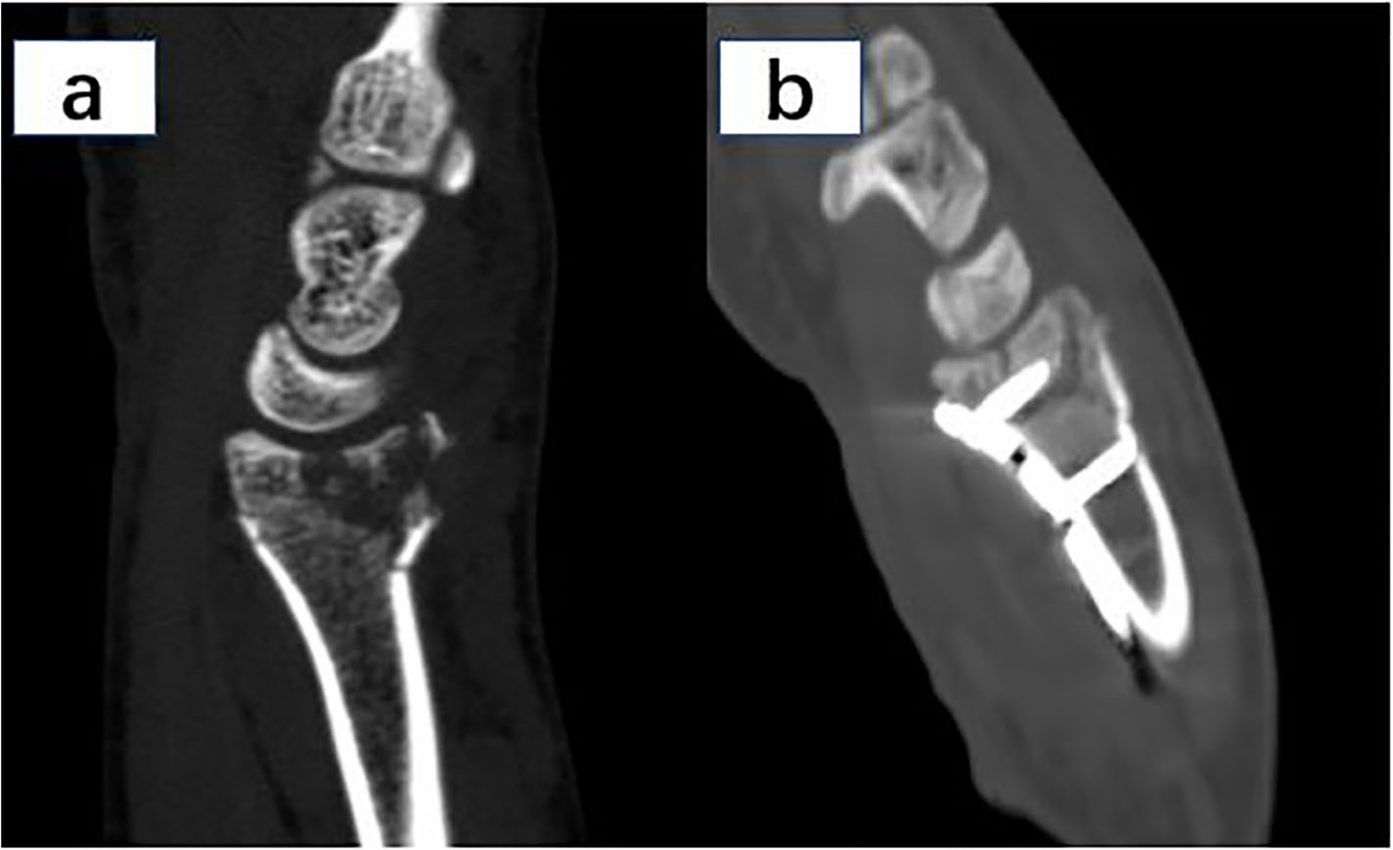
Pre- and post-operative sagittal view CT images showing the reduction and fixation of the articular fragment. a Pre-operative CT image showed the articular step and gap, as well as the dorsal comminution of the fractured distal radius; b Post-operative CT image showed the articular step and gap were well reduced
